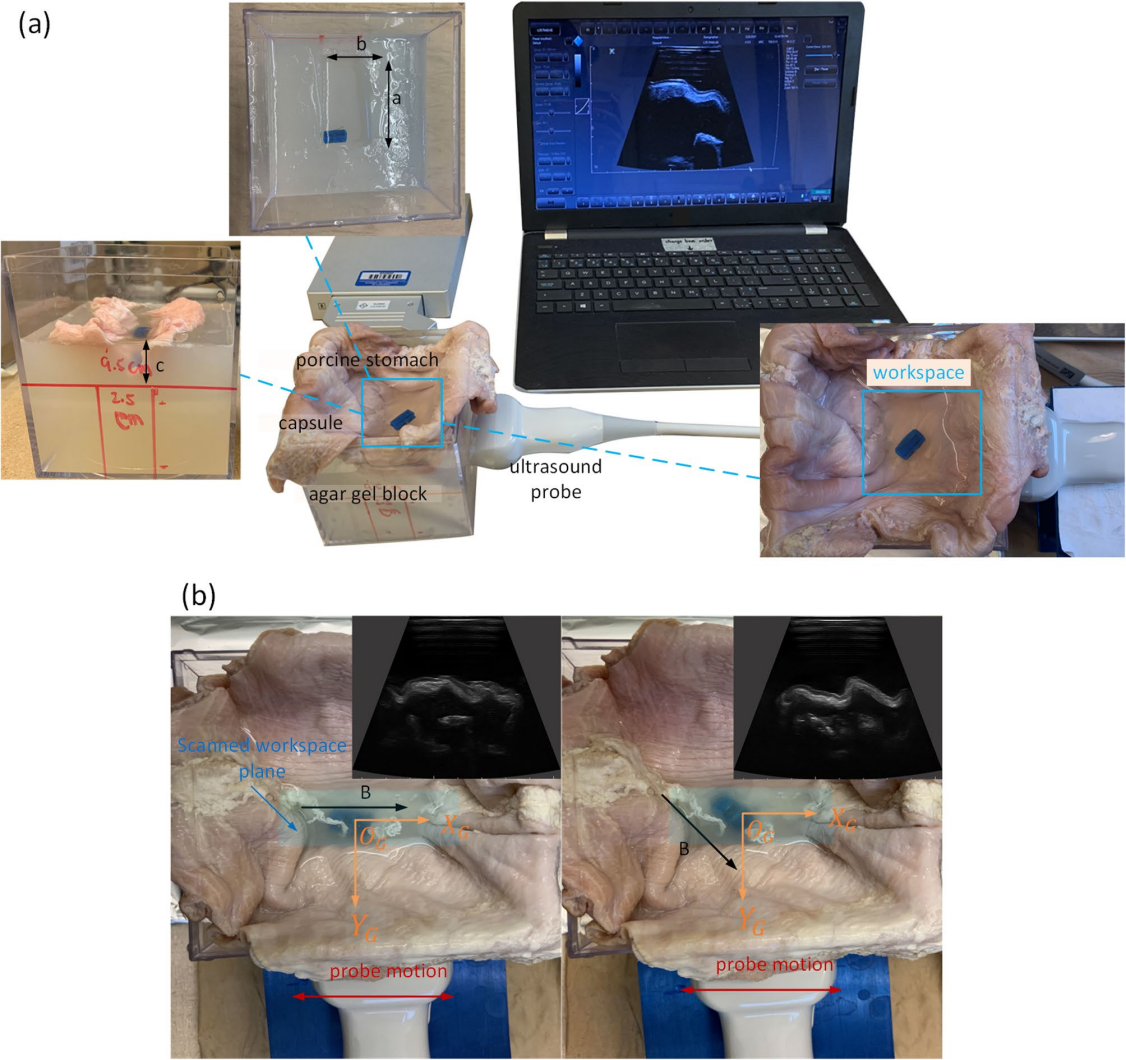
The experimental setup is depicted in (a), where the defined workspace is the embedded section of the stomach in the channel and the channel length $$a = 1.4L$$, the length of the probe, channel width $$b = 3\,cm$$ and depth $$c\ge w$$, the width of the probe. (b) shows the base dataset generation where the insets are the corresponding ultrasound images. *B* represents the external magnetic field and the red arrow indicates the translation of the probe during data collection.
